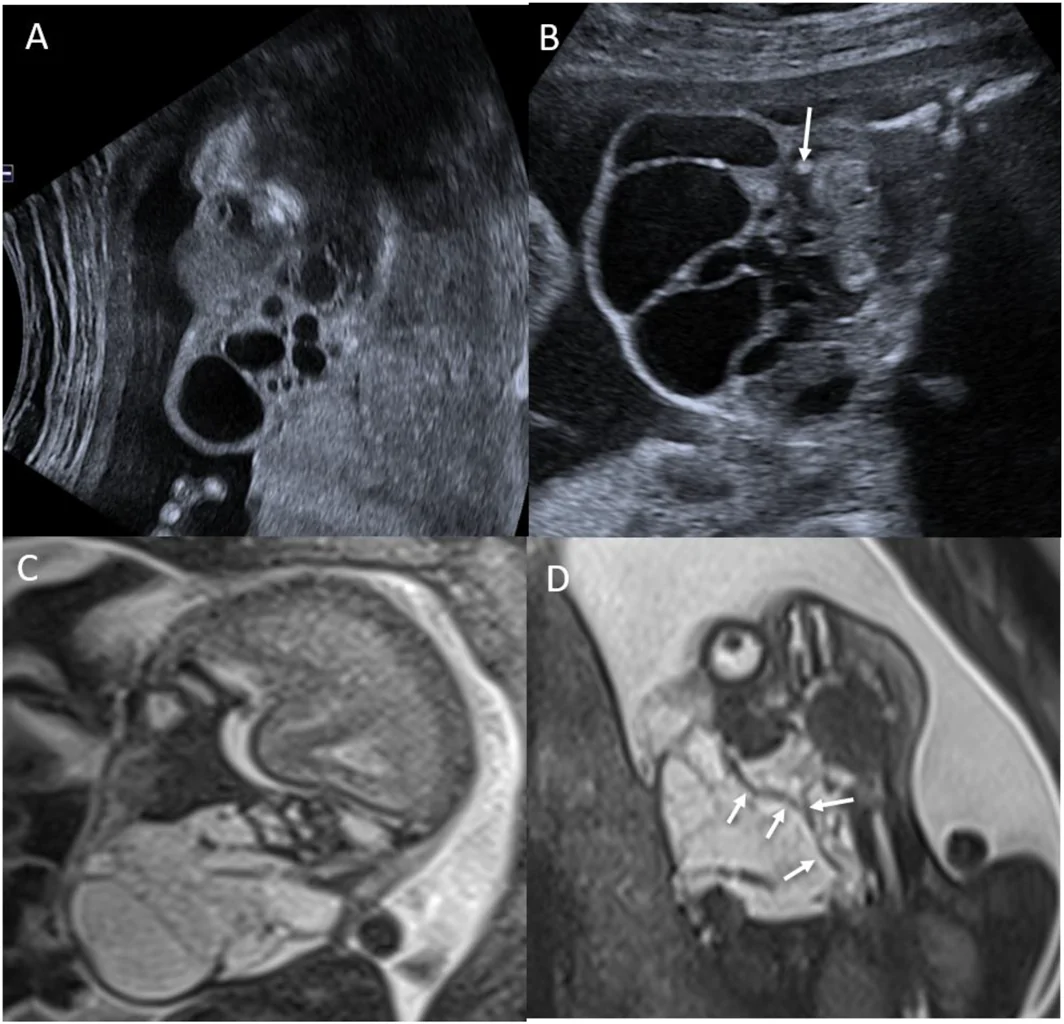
(A–D) The patient referred for extensive cervico‐facial cystic lesion suggestive of LM not included in our series due to fetal death before MST. In this case, sagittal (A) and axial (B) sonographic images performed in the second trimester showed a mainly cystic lesion with a focal echogenic spot on the axial view suggestive of calcification. On these sonographic features, cystic teratoma could not be ruled out. Sagittal (C) and coronal (D) T2‐weighted MR images showed an infiltrative lesion. Note infiltration by the LM around the main carotid artery with clear identification of the division into internal and external carotid arteries without any mass effect. This finding is highly suggestive of LM since such an absence of mass effect on the vessels would not be encountered with a teratoma.
